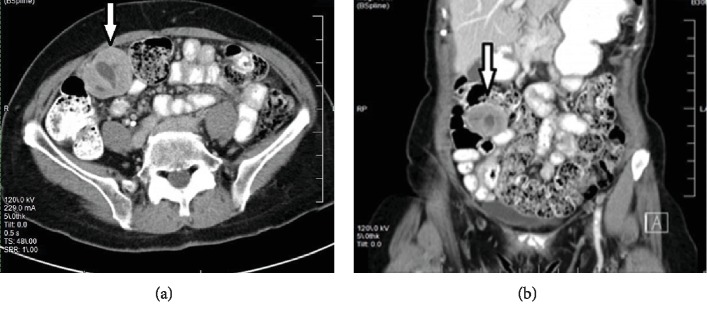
Axial (a) and coronal (b) views of CT scan of the abdomen and pelvis. Notice the right lower quadrant mass (white arrow) with low attenuation over the central region.
